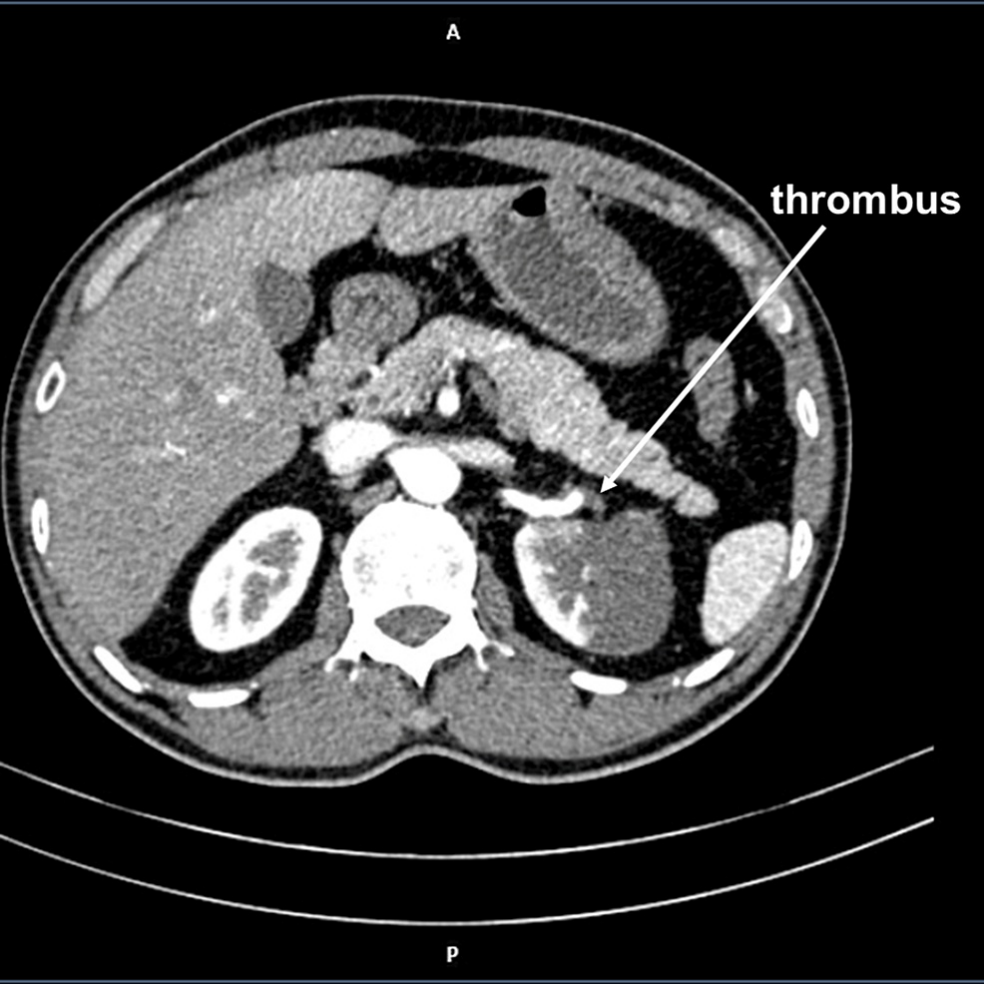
Contrast-enhanced CT scan of the abdomen showed an area of non-enhancement in the left kidney and a thrombus in the anterior segment artery
